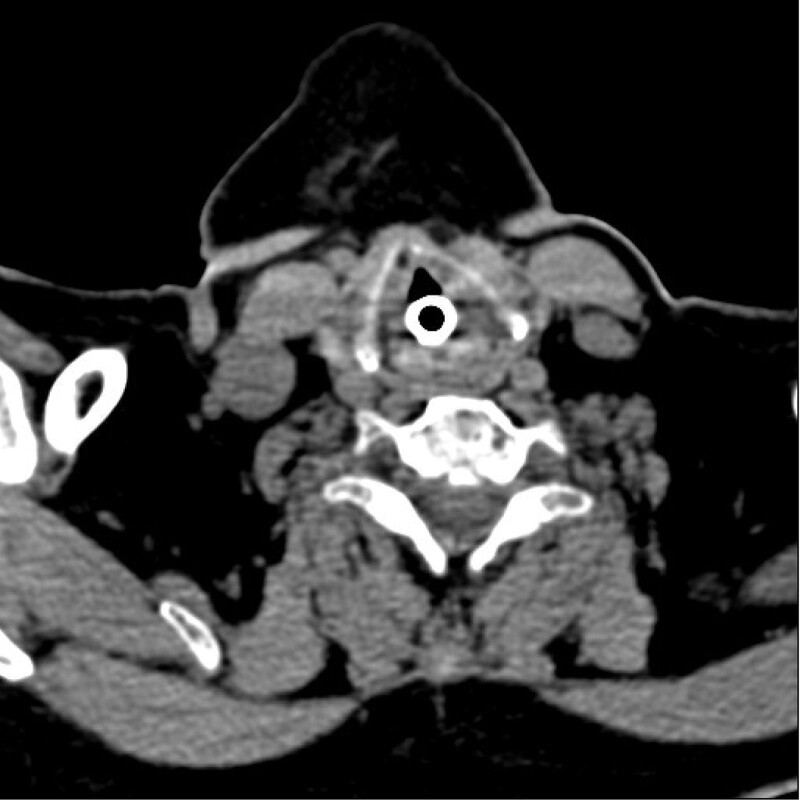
CT with tube: CT scan of the neck during tracheal tube placement showed obvious edema of the soft tissue around the glottis. CT = computerized tomography.
